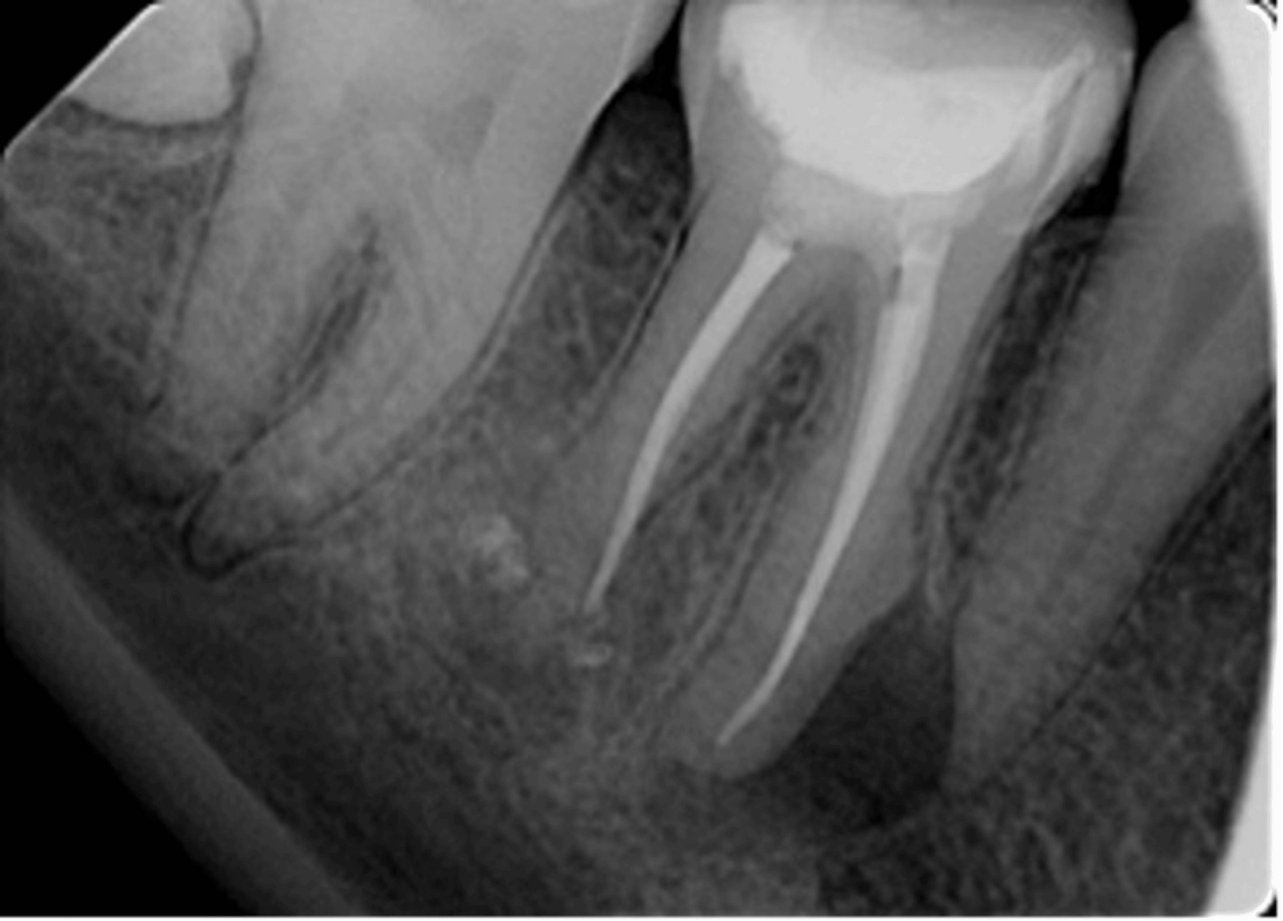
Post-endodontic retreatment periapical radiograph.
